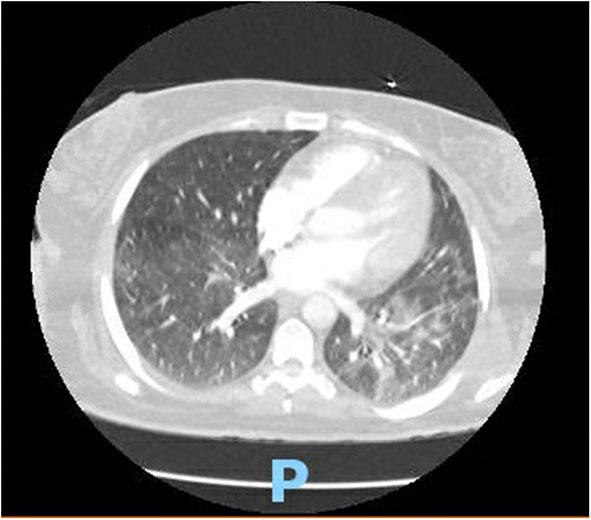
CT chest showing typical BL ground glass opacifications in lungs: a hallmark of SARS-CoV-2 infection.
